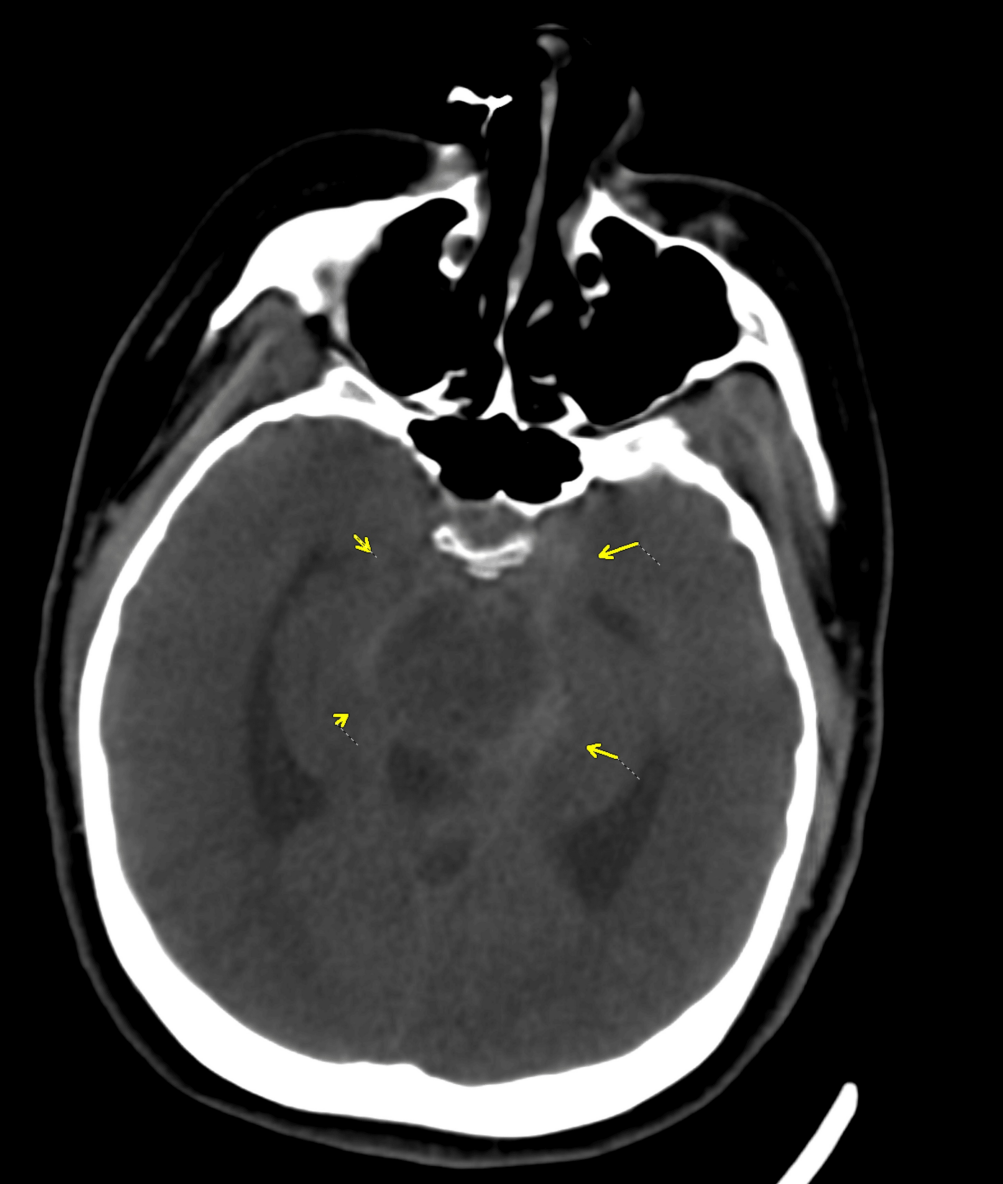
Axial non-contrast CT of the head showing diffuse subarachnoid hemorrhage (yellow arrows) within the basal cisterns. CT = computed tomography
